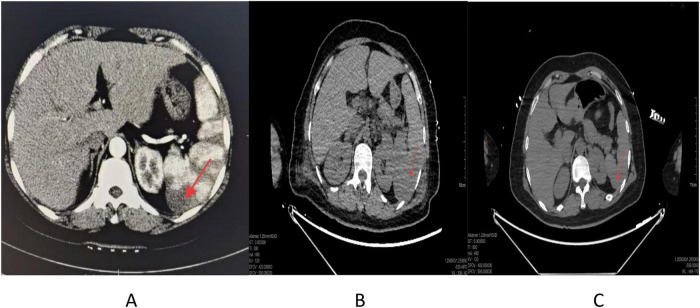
Contrast-enhanced CT images showing the evolution of splenic infarction across two hospitalizations. (A) Outside-hospital CT showing multiple wedge-shaped hypodensities in the spleen. (B) CT from the initial admission showing multifocal splenic infarcts. (C) CT during the recurrent episode showing persistent but slightly reduced infarct areas. Written informed consent for publication of radiologic images was obtained.
